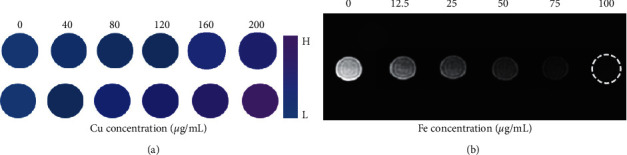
(a) The CT images of mPEG-PCL@CuFeSe_2_ NPs at different concentrations. (b) The T_2_-weighted MR images of mPEG-PCL@-CuFeSe_2_ NPs at different Fe concentrations.
